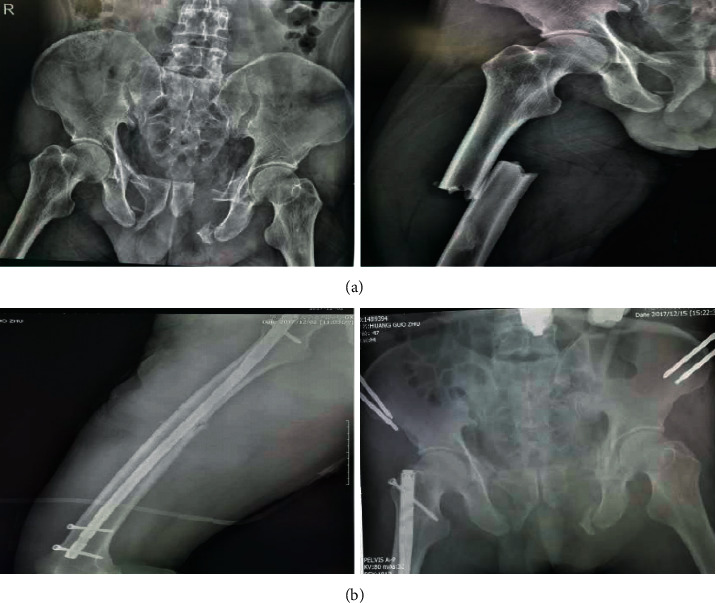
Comparison of fracture sites before and after surgery. (a) X-ray of femur and pelvis before surgery. (b) X-ray of femur and pelvis after surgery.
